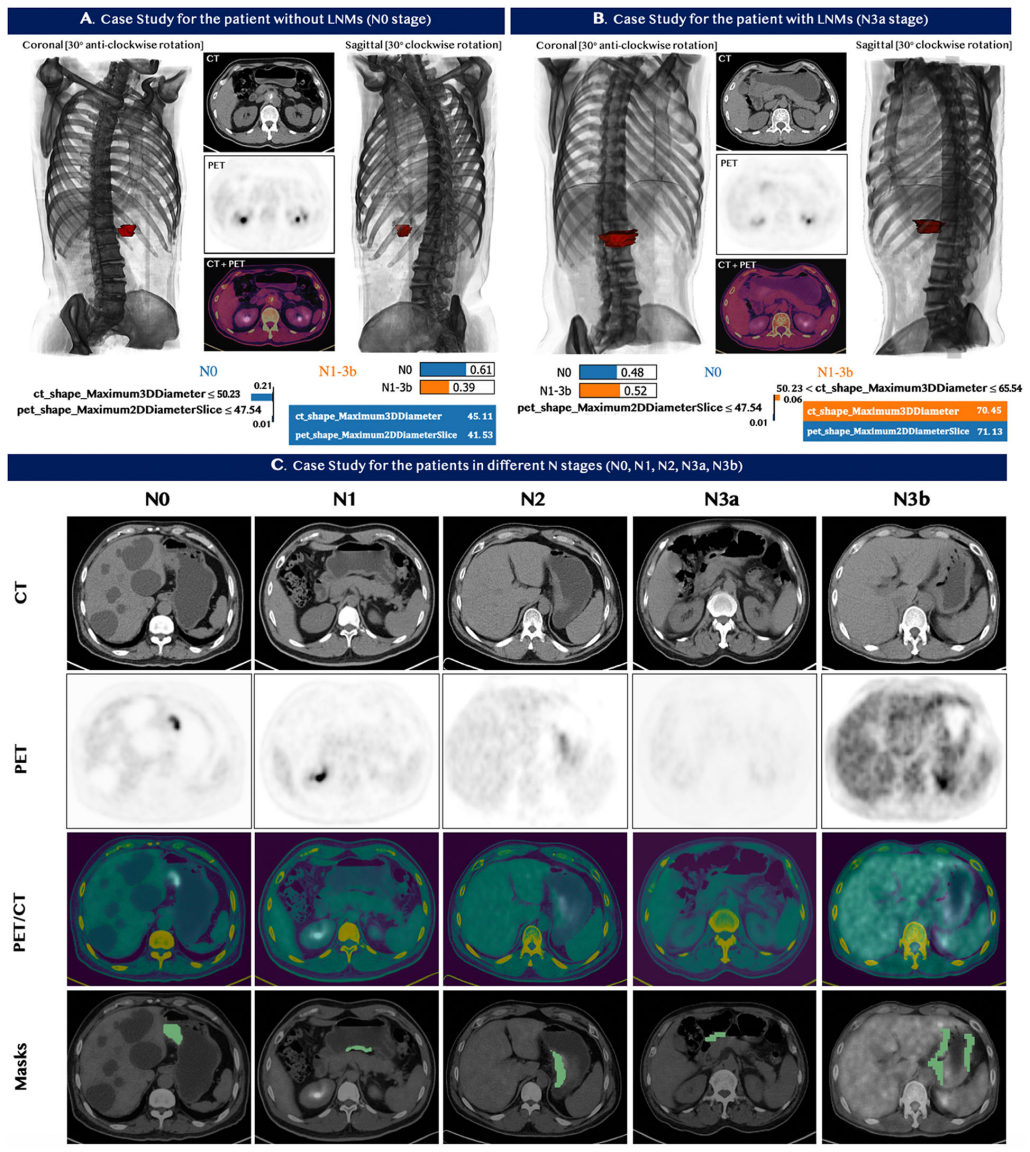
Case studies for seven patients with GC. Top Panels: (A) patient with no lymph nodes metastases. (B) patient with lymph nodes metastases. The image at the bottom of (A, B) contains the feature value of the patients and the corresponding LIME interpretation. The top left and top right sections in panel (A, B) demonstrated the 3D model constructed based on the input CT and PET images from different viewpoints, while the red section represented the tumor of the patients. Our predictive model correctly identified the status for both patients in panel (A, B). (C) Bottom Panel - Five patients with different stages N0, N1, N2, N3a, and N3b from left to right. Our machine learning model predicted the N stage of the five patients accurately. ^18^F-FDG PET/CT, however, did not detect LNMs in all five patients; and CECT also did not assess the N stages correctly.
